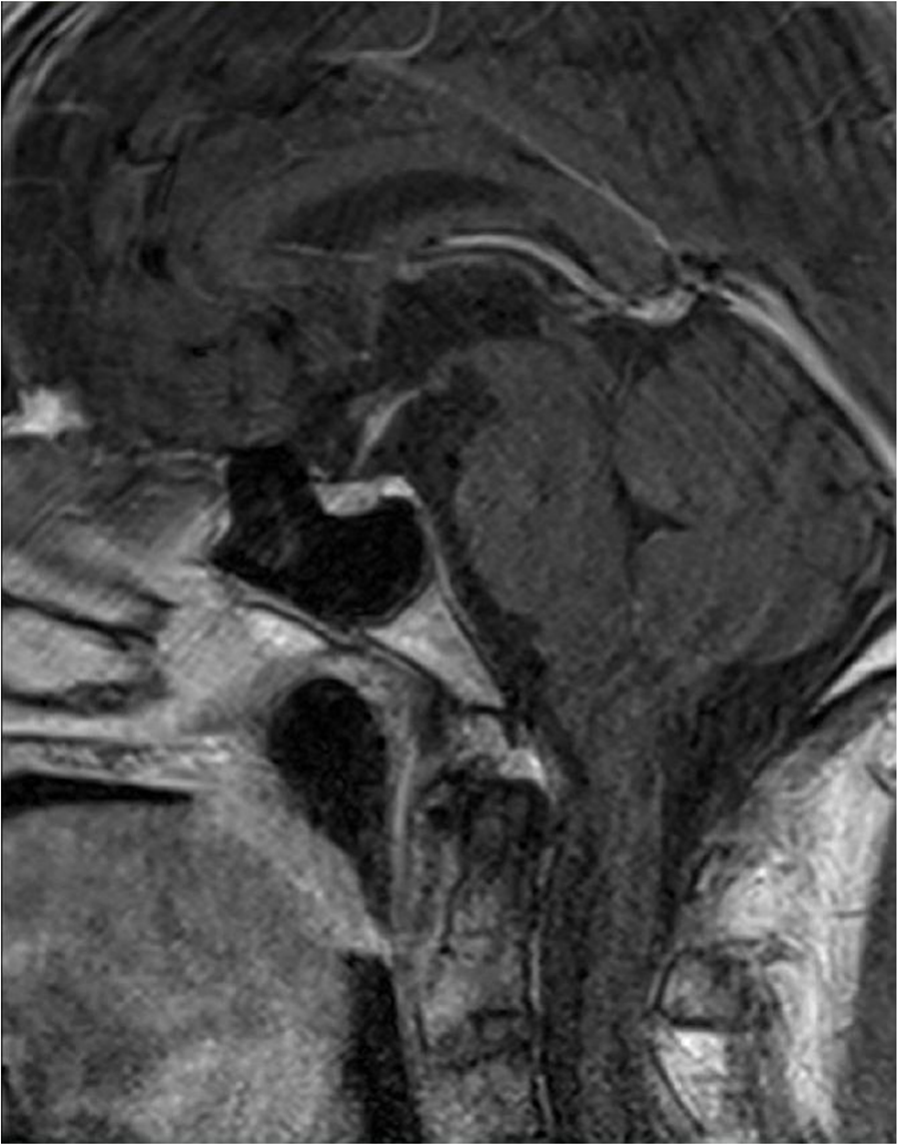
Post-contrast T2-weighted MR-image demonstrates moderately thickened infudibulum, consistent with hypophysitis
